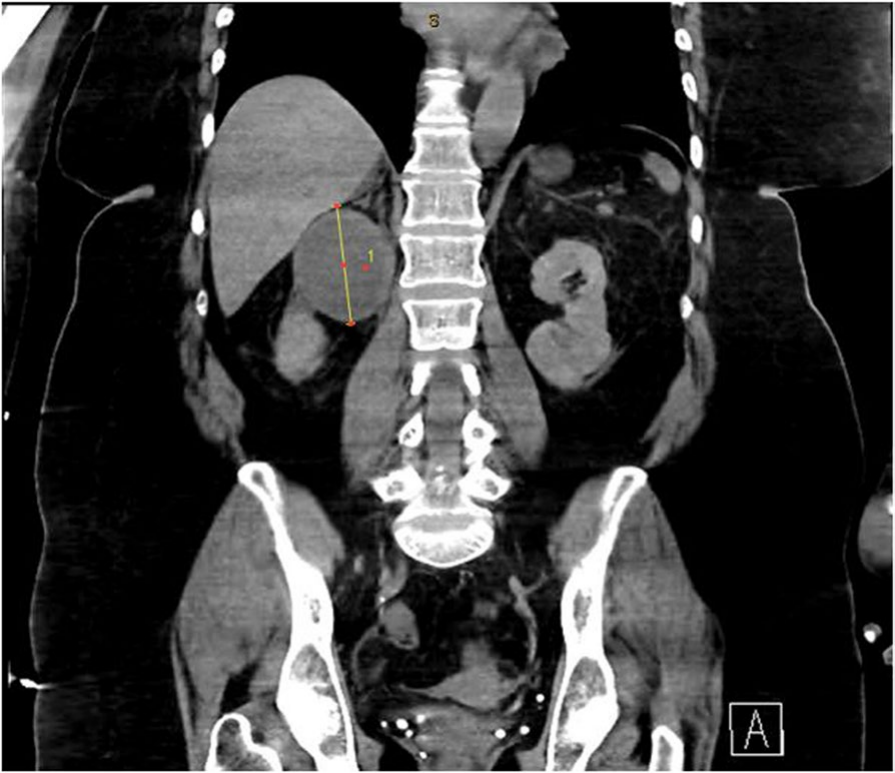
Coronal Slice of CT Abdomen revealing 6.6 m diameter right-sided adrenal mass
